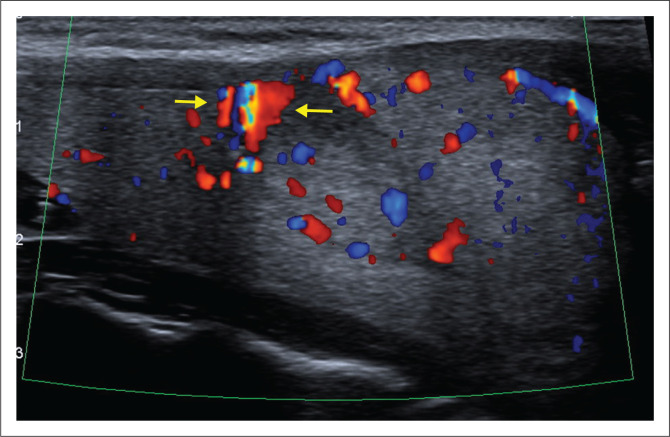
Colour flow Doppler ultrasound (longitudinal view). Focal area of increased flow (yellow arrows) superior and adjacent to the well-defined larger isoechoic nodule.
